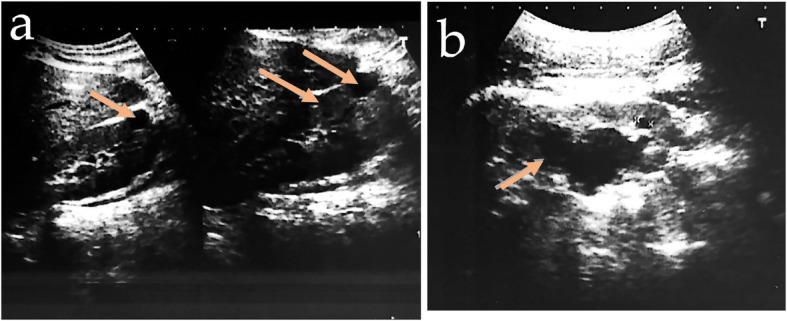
Ultrasonography showing simple renal cysts, diminished corticomedullary differentiation (a) and caliectasis (b). With the arrow are indicated the different phenotypes changes
